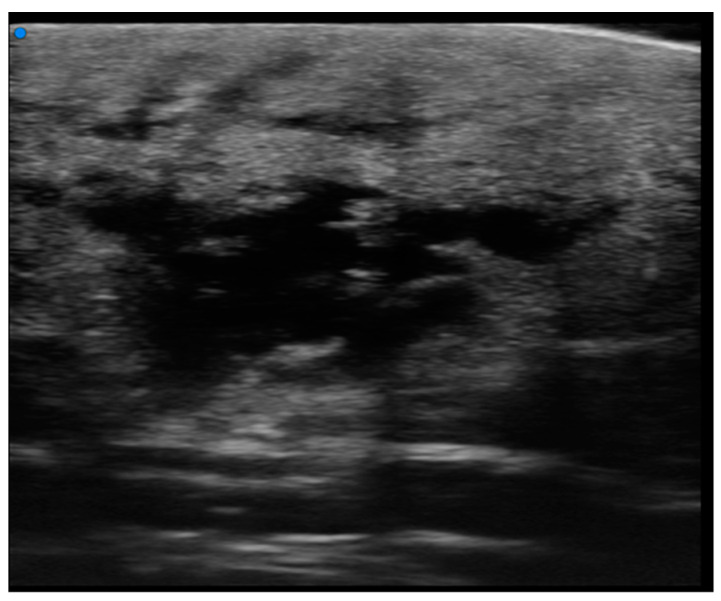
Diffuse infiltrative lesion (recurrence of B-cell lymphoma) examination performed with a very high-frequency ultrasound probe at 50 MHz in the region of the right scapula: Iso-hypoechoic lesions with nodular and multilobulated morphology and irregular margins infiltrating the epidermal and subepidermal tissue.
